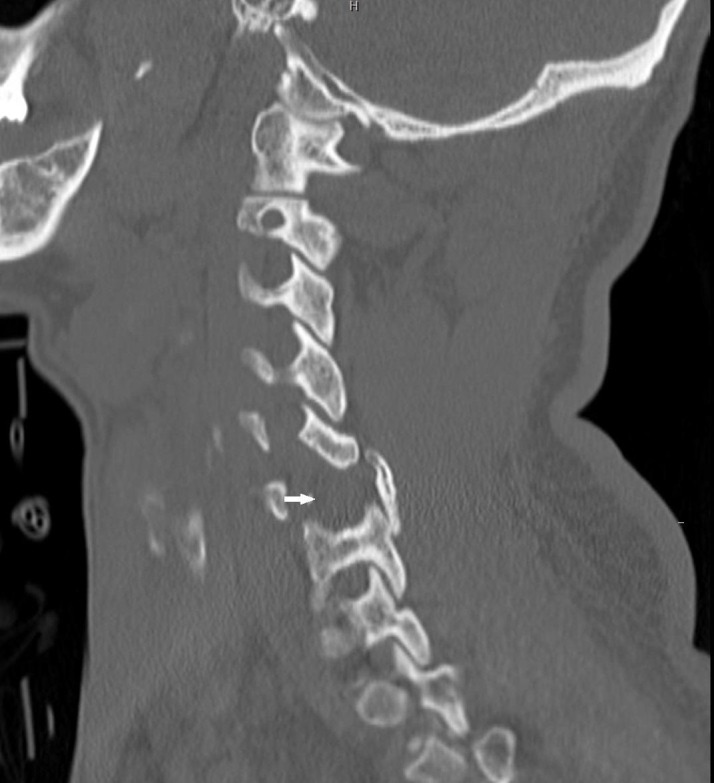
Computed Tomography Scan of the Cervical Spine Sagittal computed tomography scan of the cervical spine showing the missing C6 pedicle on the left side (white arrow)
